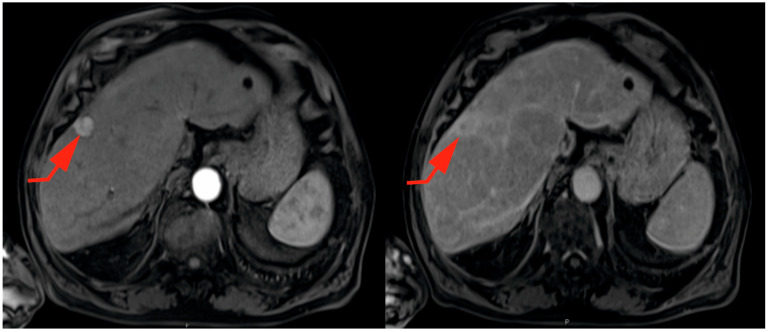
Axial image depicting the typical behavior of HCC on contrast-enhanced liver magnetic resonance imaging (MRI). It shows a hyperenhancing subcapsular focal lesion in the arterial phase (left), with isoattenuation and washout in the portal phase (right). The red arrow indicates the lesion in both phases. This is observed in a patient exhibiting signs of chronic liver disease. Additionally, a cyst is evident in segment II of the left hepatic lobe.
